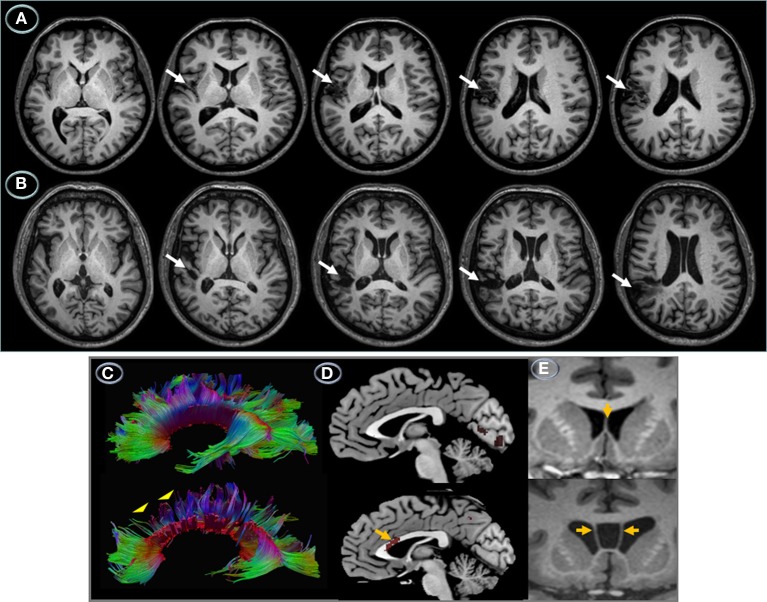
Left hemisphere structural lesions. Axial T_1_-weighted MRI showing a small infarction in the patient with transcortical motor aphasia (A) involving the left sensorimotor cortex and medial insula (white arrows). The lesion is superficial sparing the deep white matter. The MRI in the patient with conduction aphasia shows a small infarction involving the left posterior temporal gyrus and supramarginal gyrus extending deeply into the lateral ventricle wall (B) (white arrows). The left hemisphere is represented on the left side of the images. DTI, MRI, and PET of the corpus callosum. Brain imaging of the corpus callosum. Midsaggital DTI (C) and ^18^FDG-PET images (D) and coronal T_1_-weighted MRI (E) of the corpus callosum in patients RTP (top panel) and JGG (bottom panel). In RPT the corpus callosum was structurally (C) and functionally intact (D). Although RTP had an infarction in the left sensorimotor cortex, this did not result in noticeable changes in the anterior segments of the corpus callosum. The rostral body of corpus callosum in JGG shows diminished streamlines (C), bottom panel (yellow arrowheads) and decrement of metabolic activity (D), bottom panel (yellow arrow). The fact that structural and functional involvement of the anterior corpus callosum in JGG does not interrupt fibers interconnecting the damaged left temporoparietal cortex with its homologous in the right hemisphere suggests that corpus callosum involvement was unrelated to the stroke lesion. Anatomical MRIs show normal septum pellucidum (grade 0, normal) (yellow arrow) in RTP (E), top panel and an enlarged cavum septum pellucidum (grade 4, severe) (Degreef et al., 1992; DeLisi et al., 1993; Kim and Peterson, 2003) in JGG (yellow arrows) (E, bottom panel).
